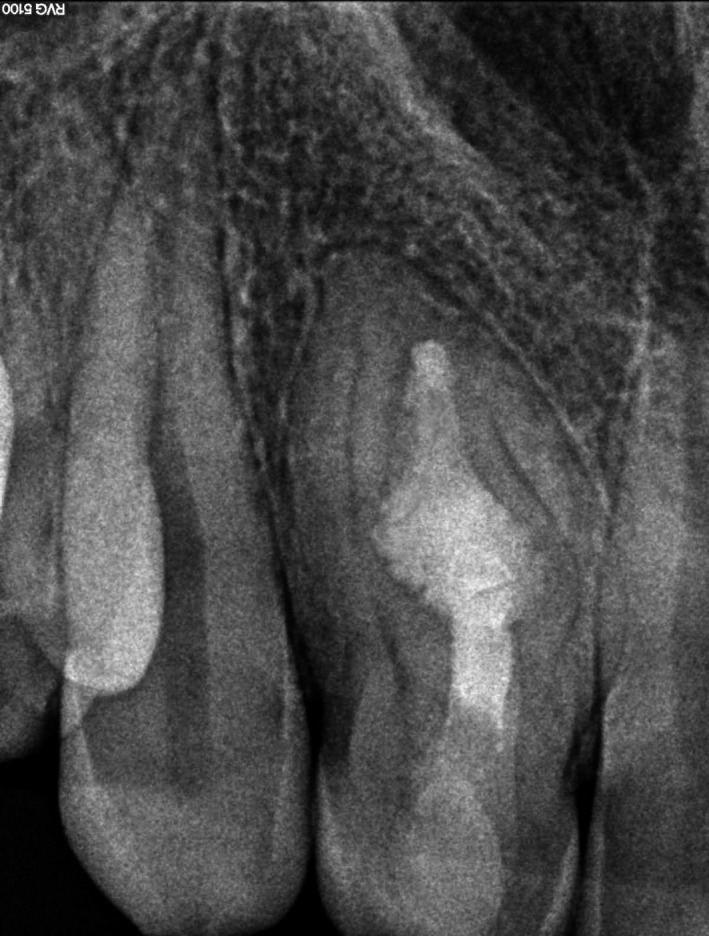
Radiographic image of tooth #12 in 24‐month follow‐up
